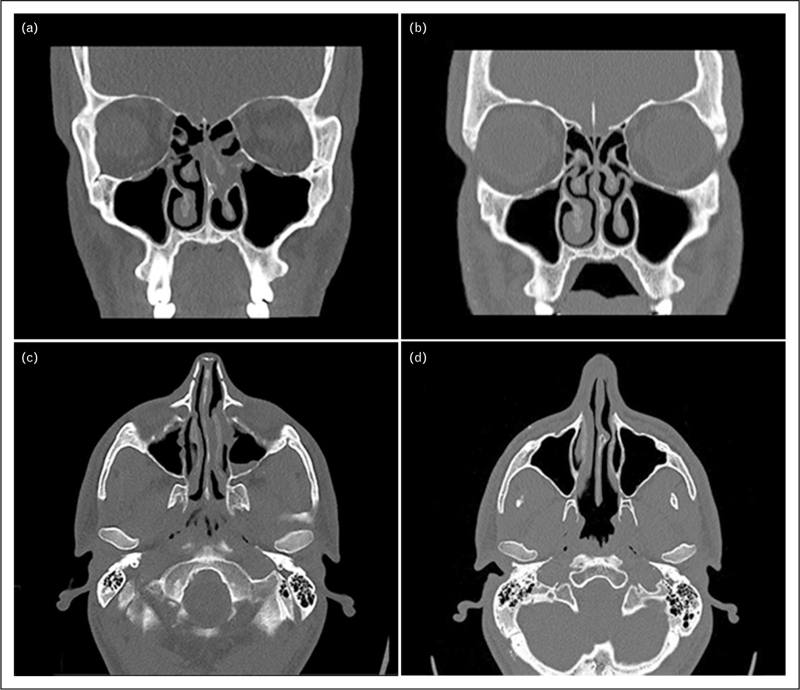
Coronal and axial CT scan performed before (a, c) and after (b, d) 16 weeks of dupilumab treatment (anti-ILRα) in a patient with CRSwNP. Images a and c show the presence of hypodense tissue with a polypoid appearance occluding the middle and upper portions of the left nasal fossa, along with signs of erosion of the ipsilateral middle turbinate. Images b and d show a significant reduction in the polypoid mass with normal pneumatization of the sinuses.
